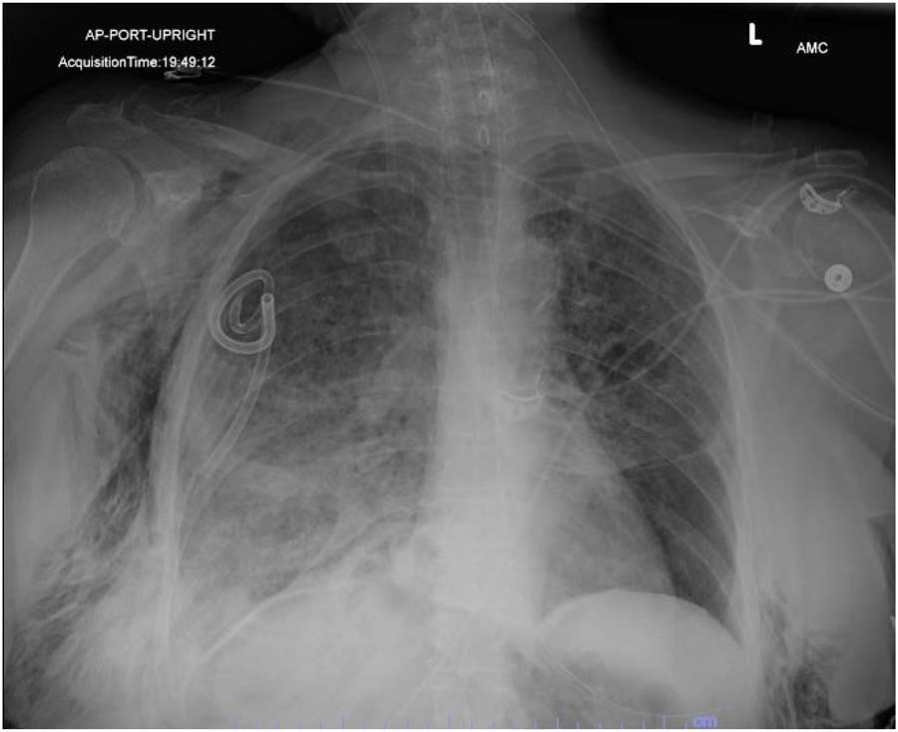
AP upright chest radiograph showing placement of right-sided chest tube and resolution of right pneumothorax.
